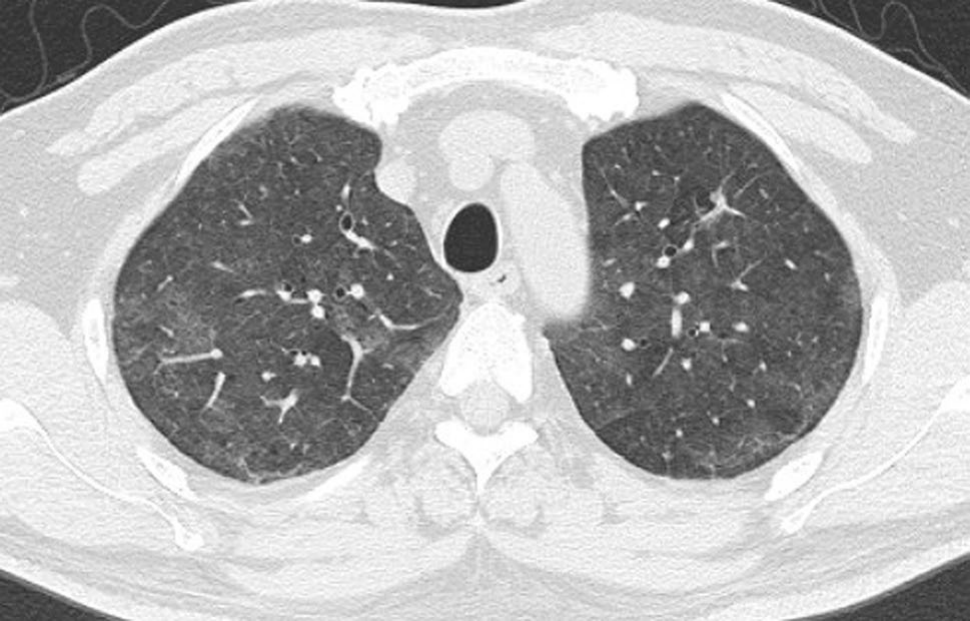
A 52-year-old man with pneumocystis pneumonia during treatment for malignant lymphoma without hematopoietic stem cell transplantation. High-resolution CT shows extensive ground-glass attenuation with a mosaic pattern. Nodules and bronchial wall thickening are not observed
